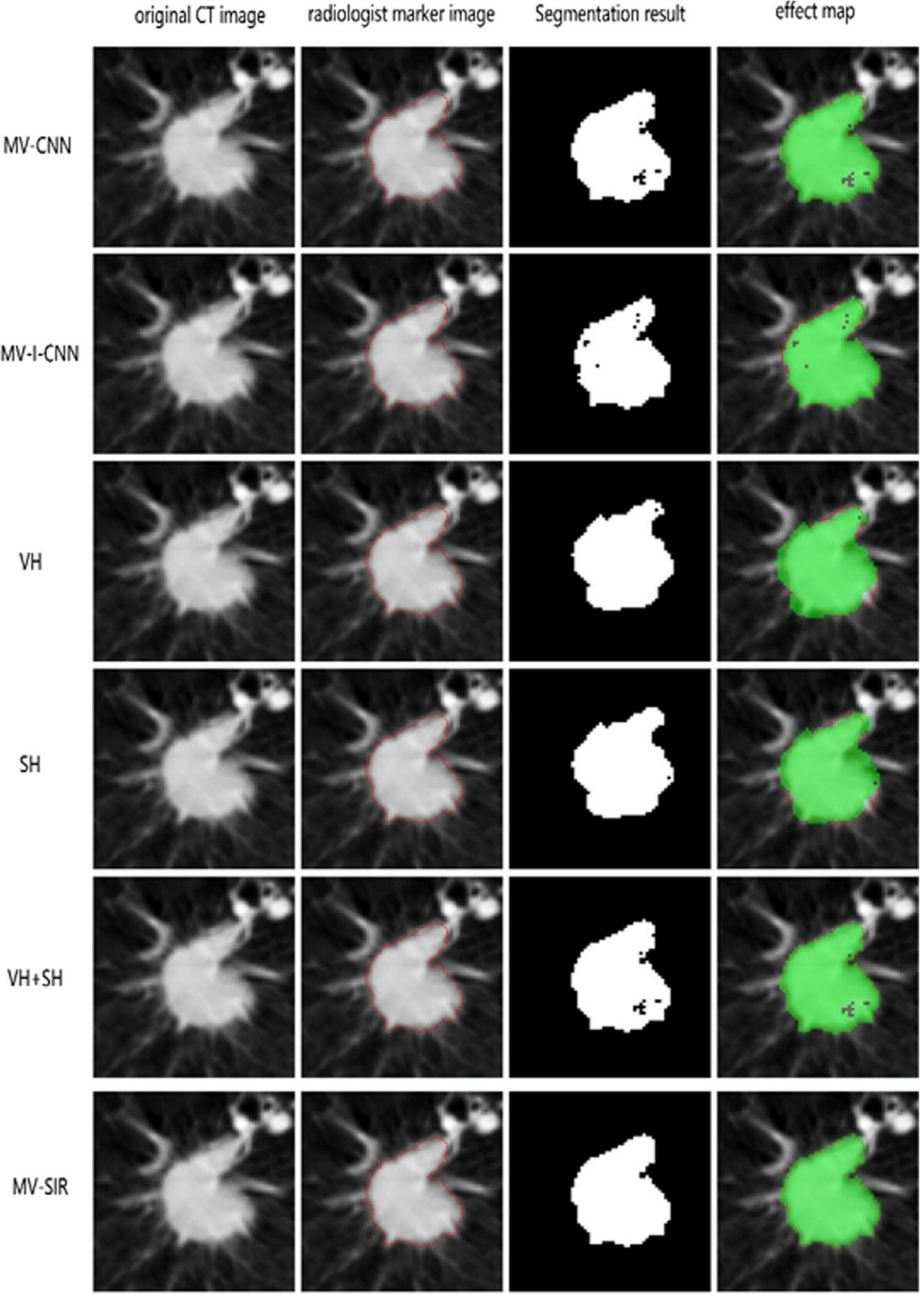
Comparison of model structures and different inputs. Columns from left to right are the original CT image, radiologist marker image, MV-SIR result, and 2D segmentation effect map. The top to bottom rows sequentially present the results of the MV-CNN, MV-I-CNN, the MV-SIR with VH input, the MV-SIR with SH input, the MV-SIR with combined VH and SH input, and secondary input MV-SIR. The 2D segmentation map can intuitively show that our model has achieved the best segmentation effect
